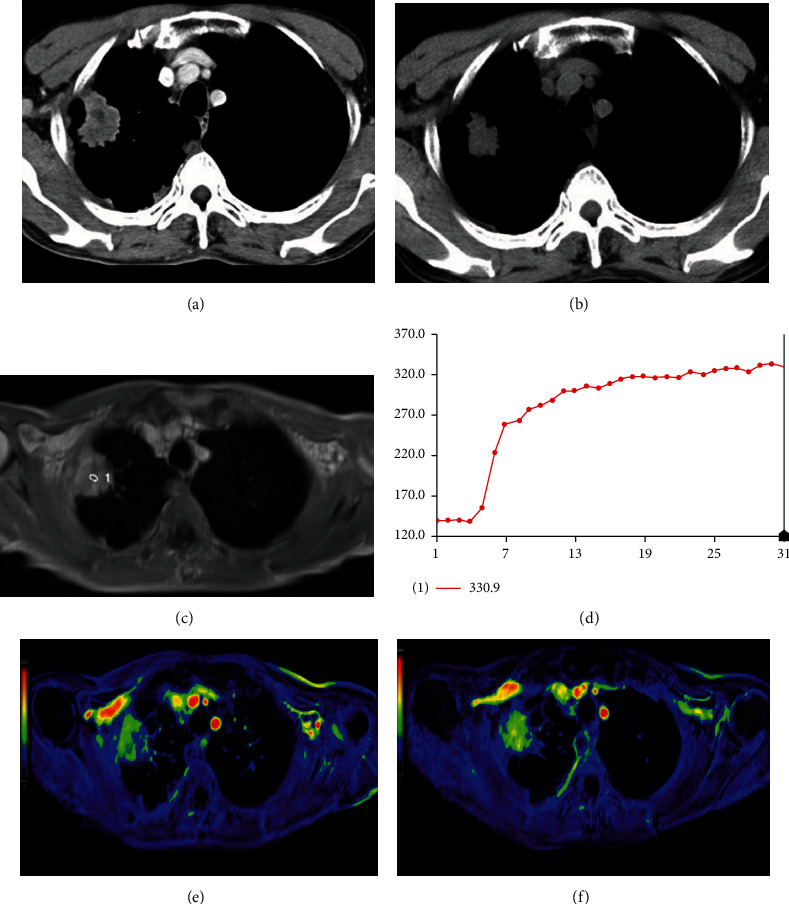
Axial CT scan of a 72-year-old male with lung adenocarcinoma at the right upper lobe before (a) and after (b) three months of chemotherapy. At the maximum transverse dimension, about 31.7% decrease of the tumor size was observed, and the patient was categorized as a responder. The DCE-MRI map (c) and TIC map (d) are shown. Images of color MR PEI mapping before (e) and after (f) one week of the first course of chemotherapy showed that there was an obvious increase in tumor perfusion (the value was 841 vs. 1100).
